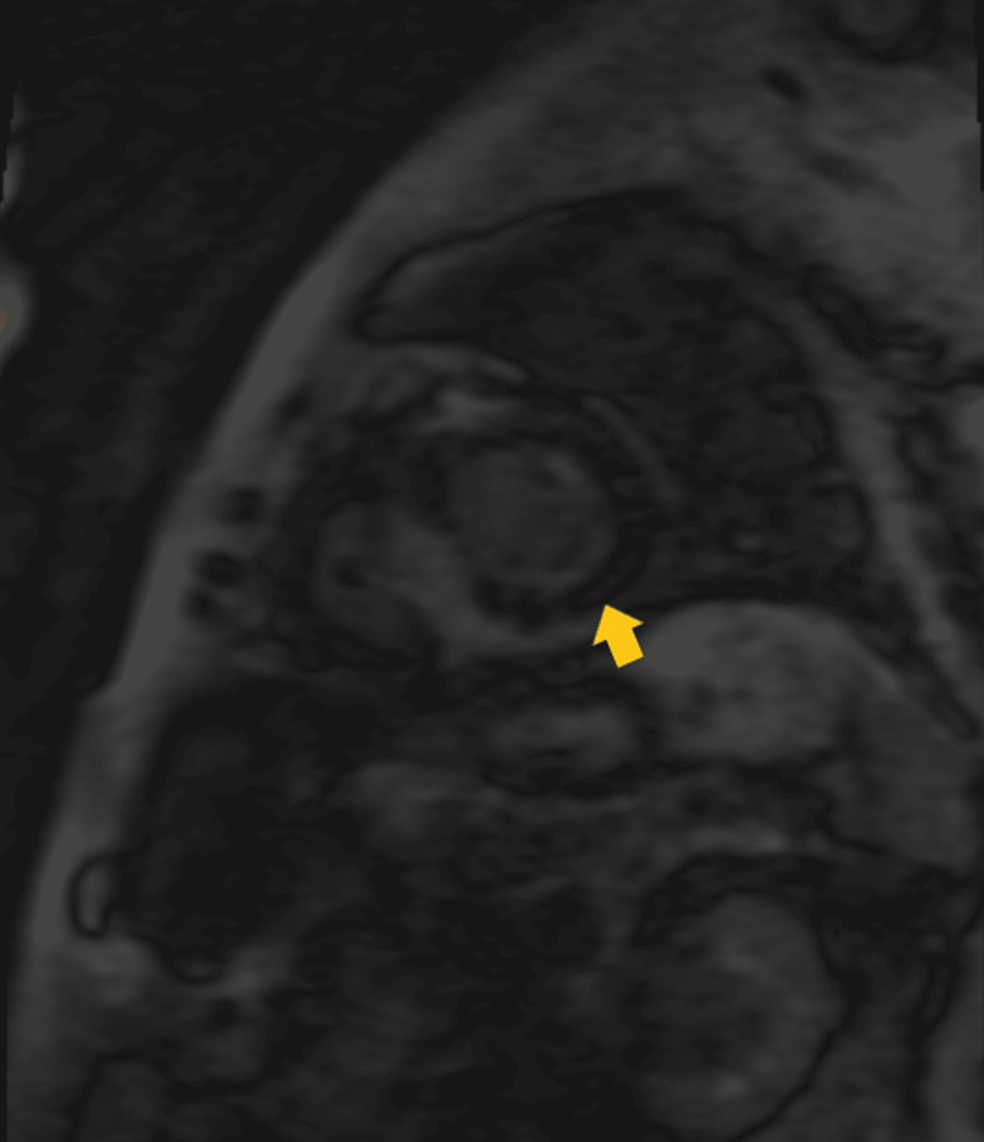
Cardiac magnetic resonance imaging (T1 Scout) with the yellow arrow showing early nulling of the myocardium and a lack of homogenous nulling.
